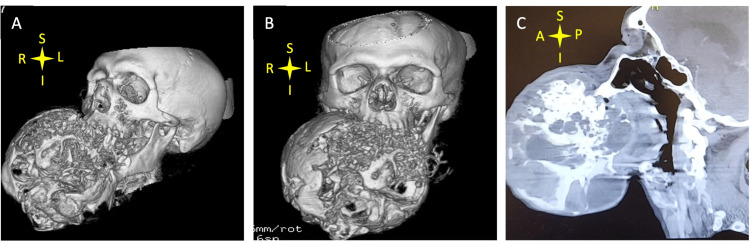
3D reconstruction of imaging (A-B); CT scan in sagittal view identifying 18x15x13cm submandibular mass (C). A: anterior; P: posterior; S: superior; I: inferior; L: left; R: right
